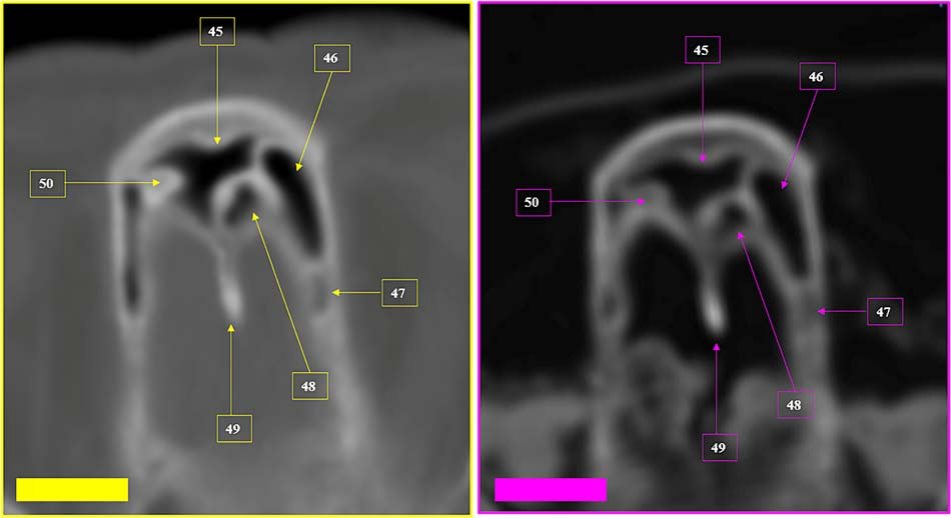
Details of the matching morphology of the frontal sinus ethmoid recess in the antemortem (left) and postmortem (right) CT scans.
